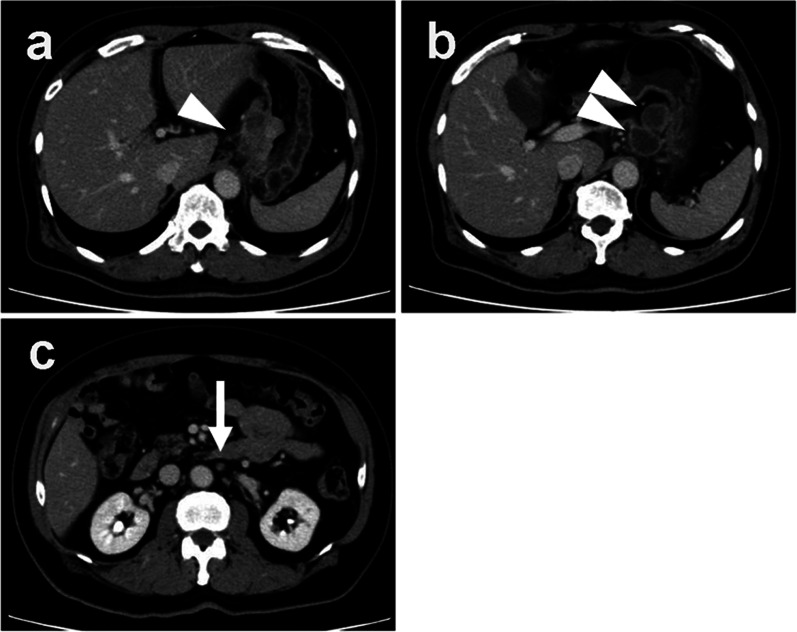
Abdominal CT. a Irregular mass at the lesser curvature of the stomach (arrowhead). b Enlarged lymph nodes at the lesser curvature of the stomach (arrowheads). c Suspected enlarged paraaortic lymph nodes (arrow)
